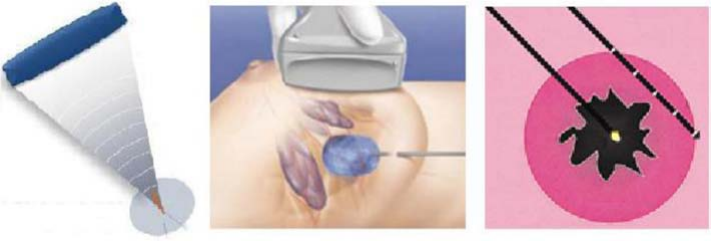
Treatment overviews: (*left*) high-intensity focused ultrasound, (*middle*) cryo-ablation and (*right*) laser ablation [23, 33, 34]
